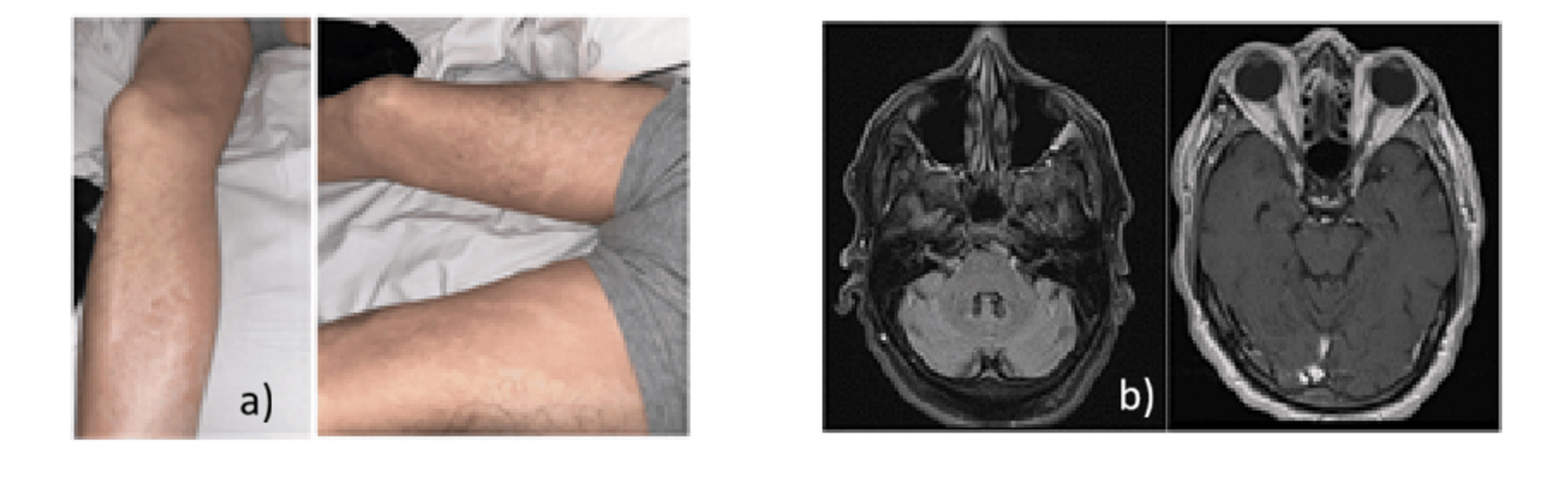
Erythematous rash in the lower extremities (a) and MRI T2 FLAIR (left) and T1 (right) (b) The patient exhibits an erythematous rash, which is attributed to syphilis (interestingly, there was no rash on the palms of the hands). The brain MRI did not show any cranial nerve or meningeal enhancement. FLAIR: fluid-attenuated inversion recovery
